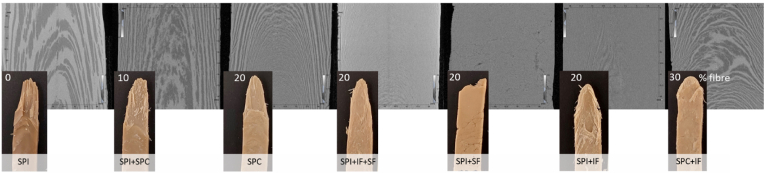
Wedges and X-Ray reconstructions of seven samples of soy protein with varying fibre content.
